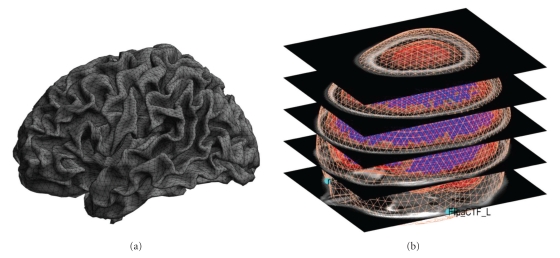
Template meshes used for distributed source imaging. (a) “Normal” cortical template mesh (8196 vertices), left view. The triangular grid shows the representation of the cortical surface used by SPM. (b) All template meshes (cortex, inner skull, outer skull, and scalp) superimposed on the template MRI. Default fiducial locations associated with the template anatomy are displayed in light blue.
